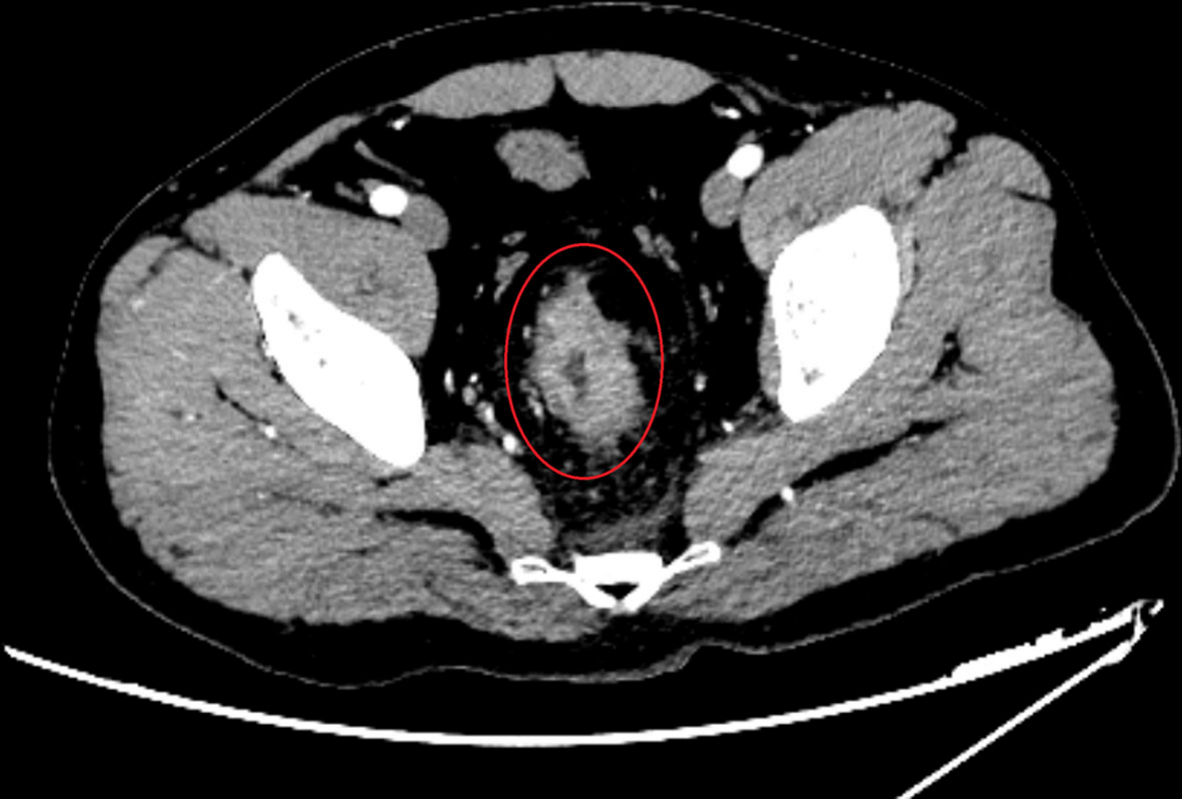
Imaging examinations performed before surgery. Enhanced CT scans on March 21, 2020 of abdomen revealed that occupying lesions in the middle and upper rectum, the intestinal lumen was narrowed, and the serosal layer was hairy. After enhancement, the lesion was uneven and enhanced, and the length of the lesion was about 5.7 cm, considering that was rectal cancer (T4aN1M0).
